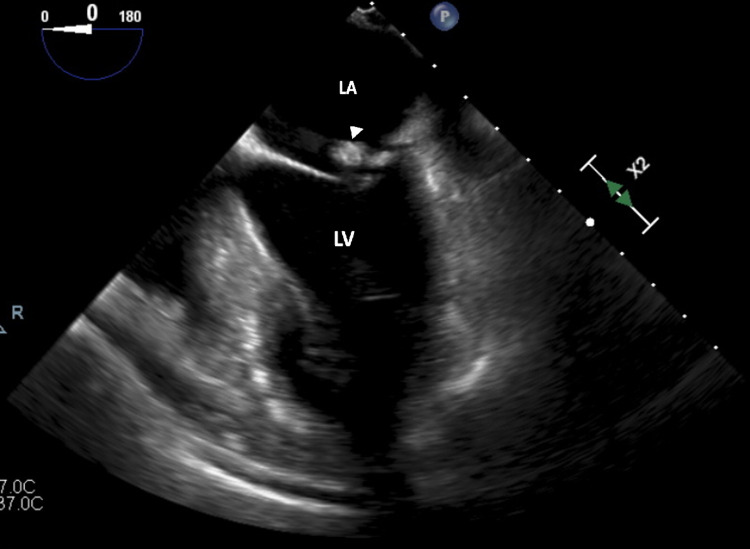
Transesophageal mid-esophageal view at zero-degree orientation showing the annular density CMAC (arrowhead). Though the orientation has changed, chamber labeling remains the same. LA = left atrium, LV= left ventricle, CMAC = caseous mitral annular calcification.
